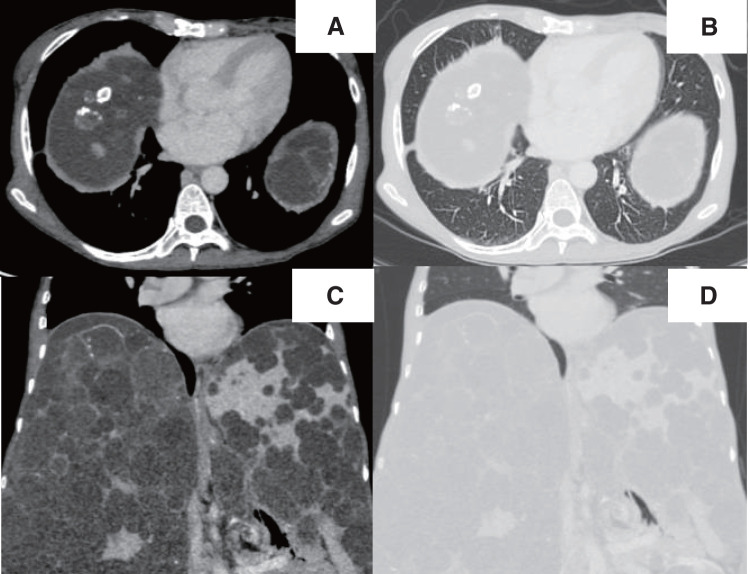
Contrast-enhanced CT findings. Before liver transplantation, CT revealed that the right diaphragm was being pushed up by multiple hepatic cysts. Axial view using a soft tissue window setting (A) and lung window setting (B), and coronal view using a soft tissue window setting (C) and lung window setting (D). CT, computed tomography
